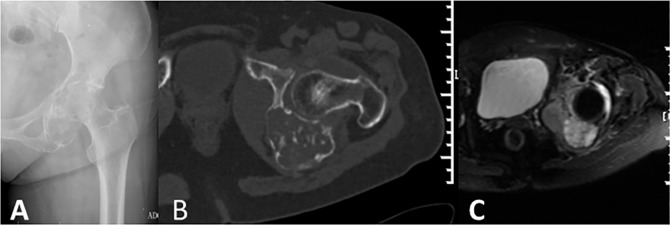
Dedifferentiated chondrosarcoma of the left pelvis in a 59-year-old woman (Case 12). (A). Anteroposterior radiograph demonstrated unilaminar benign periosteal reaction and low-grade chondrosarcoma. (B).CT showed expansive osteolytic bone destruction with typical chondral matrix mineralization and extra-osseous unmineralized soft tissue a mass cluing dedifferentiated component. (C). Fat-suppressed coronal T2-weighted image showed clear demarcation between reduced signal intensity (dedifferentiated component) and chondral signal intensity. The preoperative needle biopsy was accurate based on MRI.
